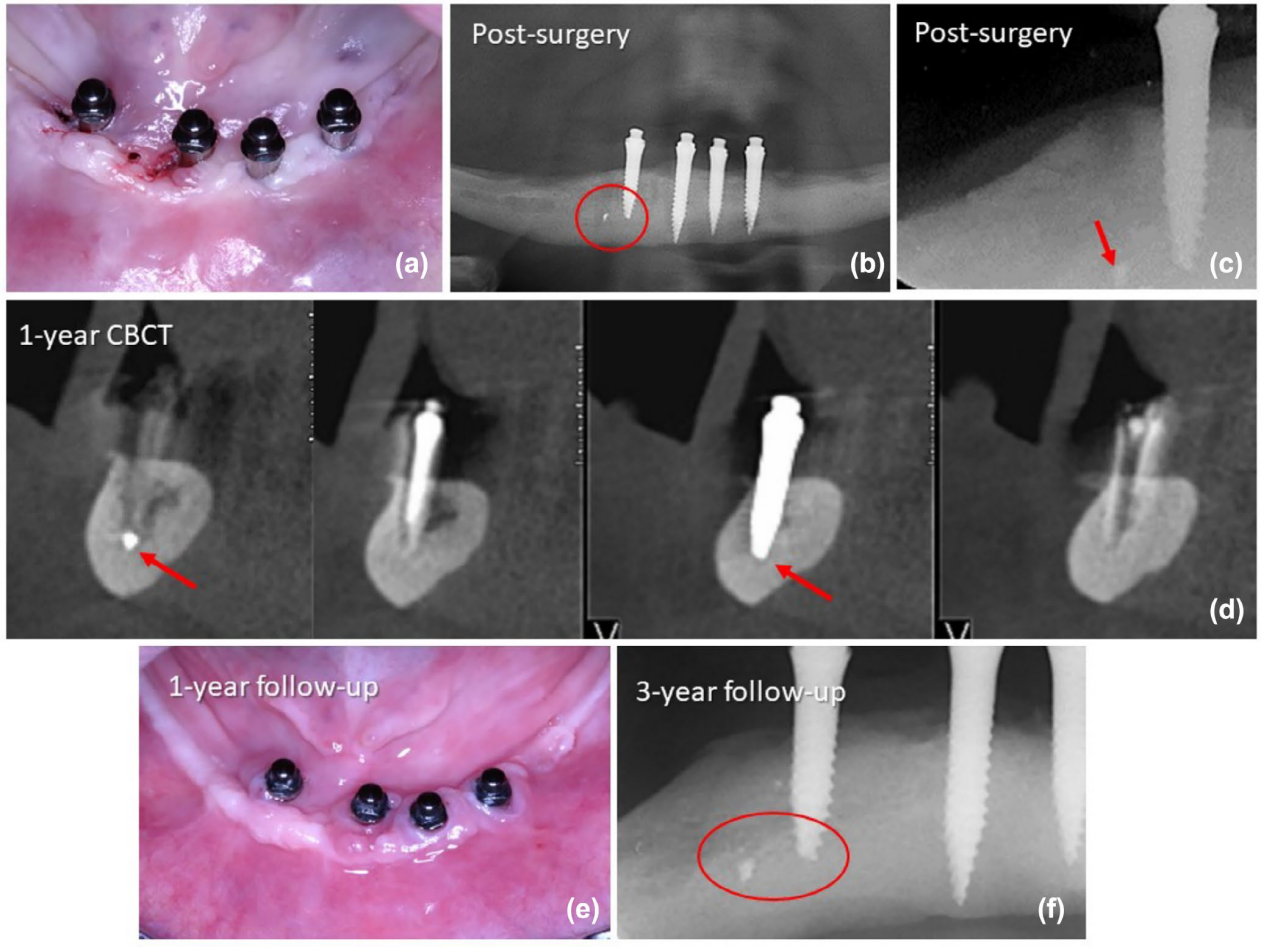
(a) Flapless transoperative view showing the first drilled site and the final position of the mini implants; (b) Post‐surgery panoramic radiograph showing a radiopaque image distal to the lateral right implant; (c) Post‐surgical periapical radiograph of mini implant and the radiolucent image of the first drilled site with the fractured fragment; (d) CBCT at the 1‐year follow‐up showing the satisfactory osseointegration of the implant and the fractured fragment. (e) Intra oral view at the 1‐year follow‐up; (f) Periapical radiograph of the right‐sided implants at the 3‐year follow up, showing the complete osseointegration around the implant and the fractured fragment.
